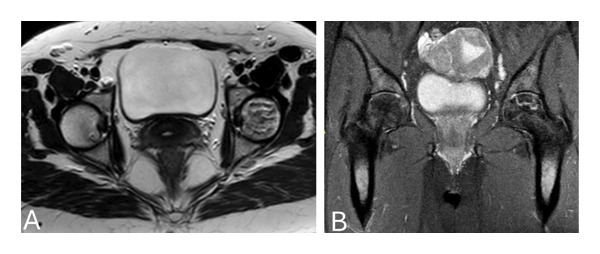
Hip MRI showing osteonecrosis in both femoral heads in axial (A) and coronal views (B).
